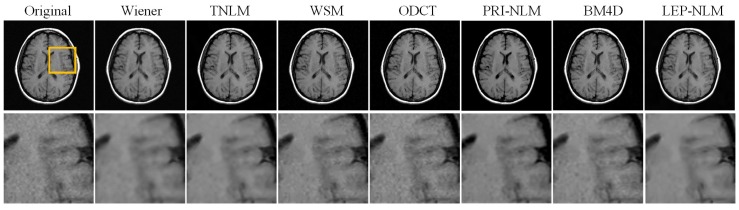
The restoration results of a real T1-weighted brain MR image by the various denoising filters. From top to bottom: the original MR images and the corresponding enlarged portions of ROIs marked with the yellow box in the original images.
